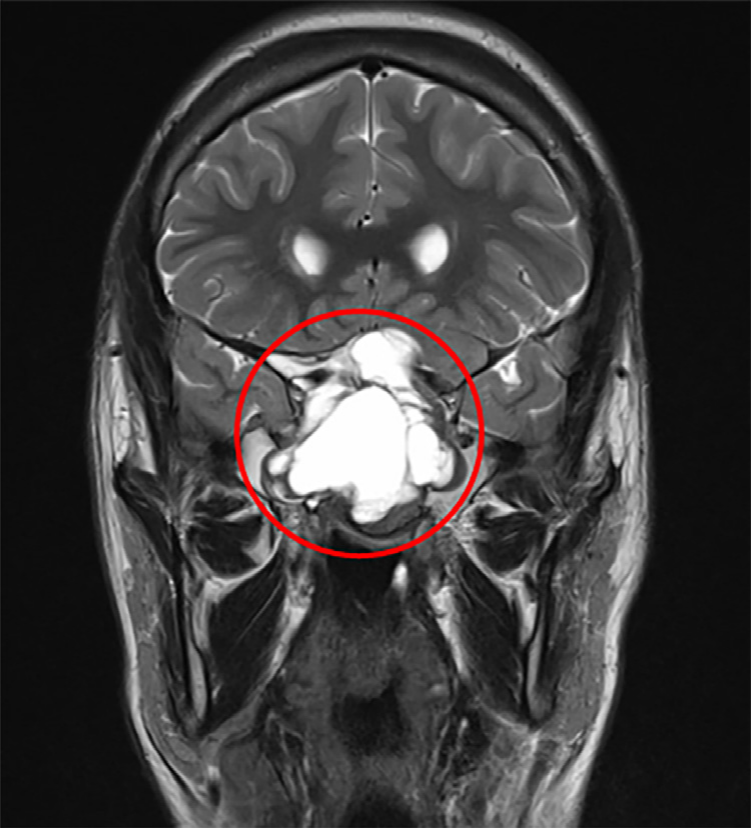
Coronal T2-w sequence on 3T MRI showed hyperintense lobulated mass (red circle) in the clivus with mass effect on the adjacent structures.
